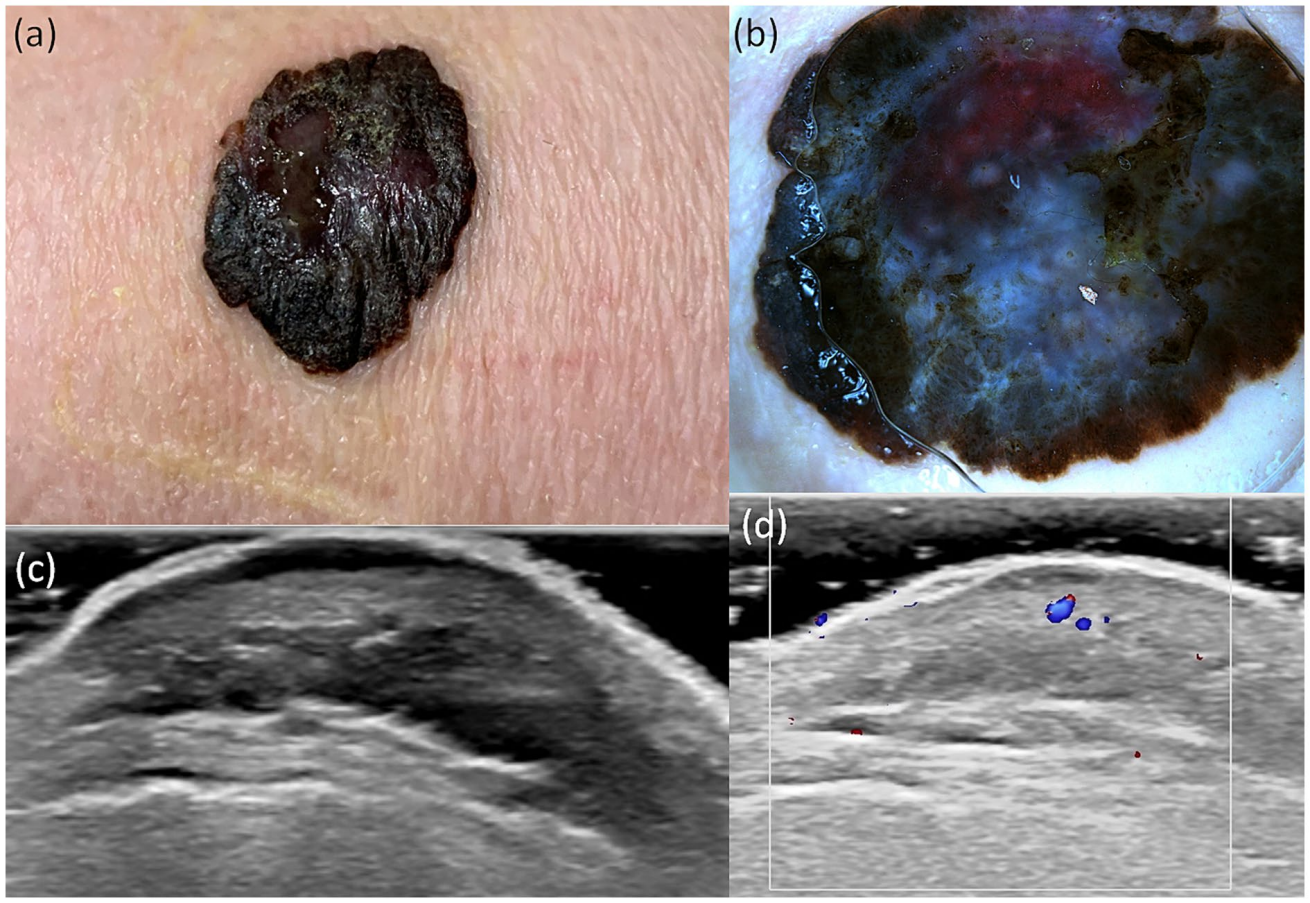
Clinical picture of SSM (A). Dermatoscopy shows the presence of an atypical network, irregular hyperpigmented areas, irregular blotch, pseudopods, atypical vesseles, and blue–black color (B). US shows a hypoechoic, oval, ulcerated, well-defined lesion that reaches the dermis (C). Doppler mode shows an increase in tumor vascularity (D).
